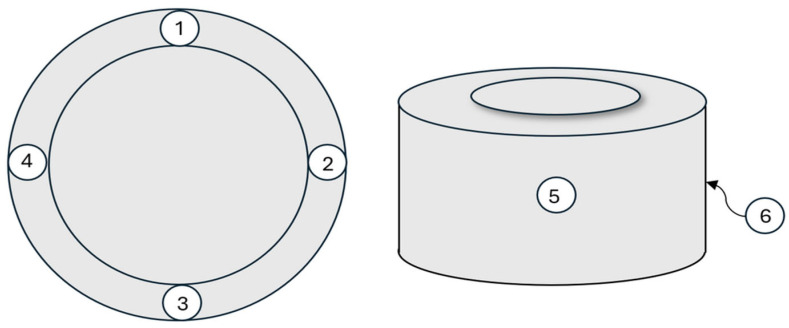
Showing the points where ultrasonic wave velocity measurements were taken.
